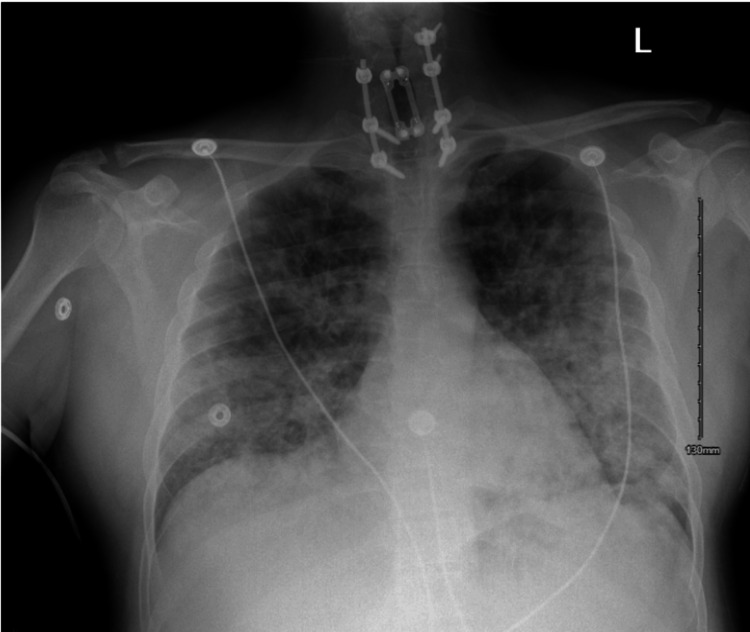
Anteroposterior chest X-ray showing diffuse, bilateral patchy alveolar infiltrates.
